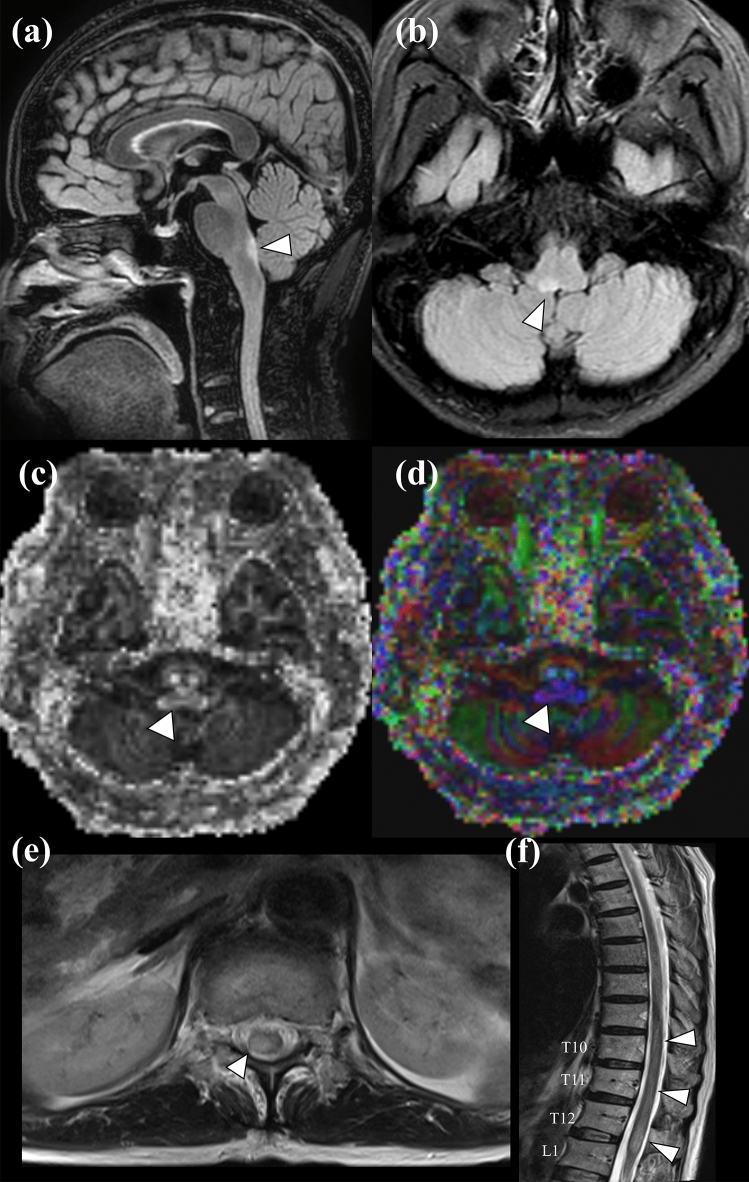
Representative patient with NMOSD with AQP4-IgG. A 57-year-old woman presented with intractable vomiting and gait disturbance (EDSS = 2; periependymal area of 4th ventricle: FA = 0.665, AD = 0.000609; periependymal area of 3rd ventricle: FA = 0.529, AD = 0.0011; periependymal area of left lateral ventricle: FA = 0.785, AD = 0.0008; and periependymal area of right lateral ventricle: FA = 0.780, AD = 0.00086). Note the periependymal T2 hyperintense lesion of right posterior medulla (or involving area postrema) on FLAIR image (*arrowhead*) (a) sagittal view, (b) axial view, as well as decreased (*dark area*) FA values on the (c) grayscale FA map, and heterogeneous colors on the (d) FA color-coded map at corresponding lesions (*arrowhead*). The patient also had an extensive, eccentric T2 hyperintense spinal cord lesion at the T10-L1 level (*arrowhead*) (e) axial view, (f) sagittal view.
